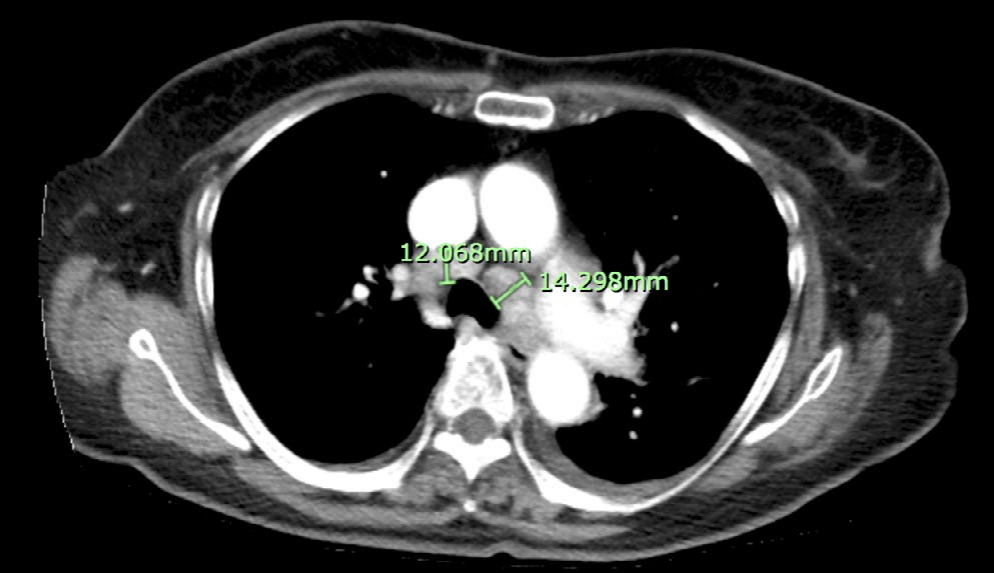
A follow‐up chest, abdomen, and pelvis CT scan with contrast showing multiple enlarged mediastinal lymph nodes without any evidence of a hilar or lung parenchymal mass
